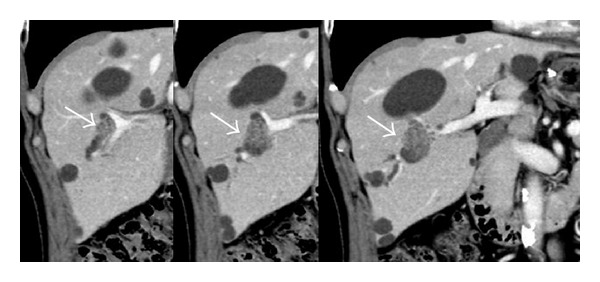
Representative images of intraductal papillary neoplasm of the bile duct on computed tomography. Localized bile duct dilatation and an intraductal mass are shown (arrows).
